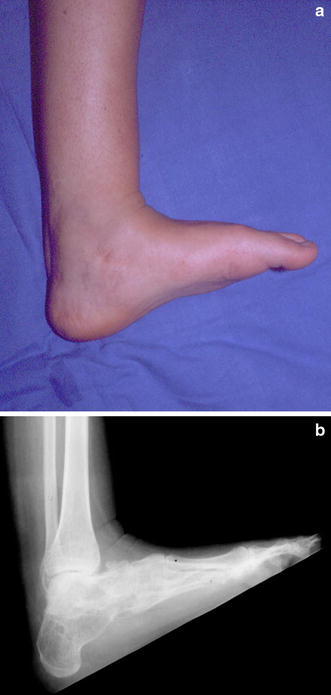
25-year-old female (patient n° 20). Preoperative photograph (a) and plain X-ray (b) of the poliomyelitic foot with vertical calcaneus deformity (maximum dorsal flexion)
